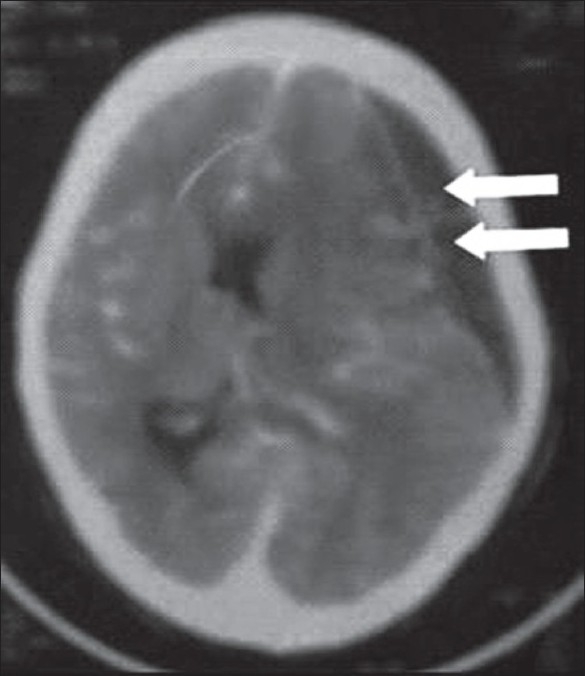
CT Image showing bilateral ophthalmic vein thrombosis with cavernous sinus thrombosis
